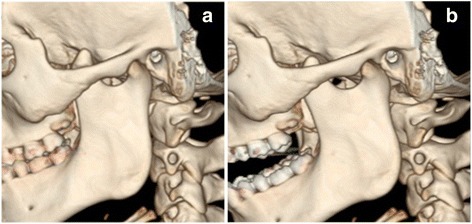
CT scan reconstructions of enlarged left coronoid process in a closed and b open jaw position
